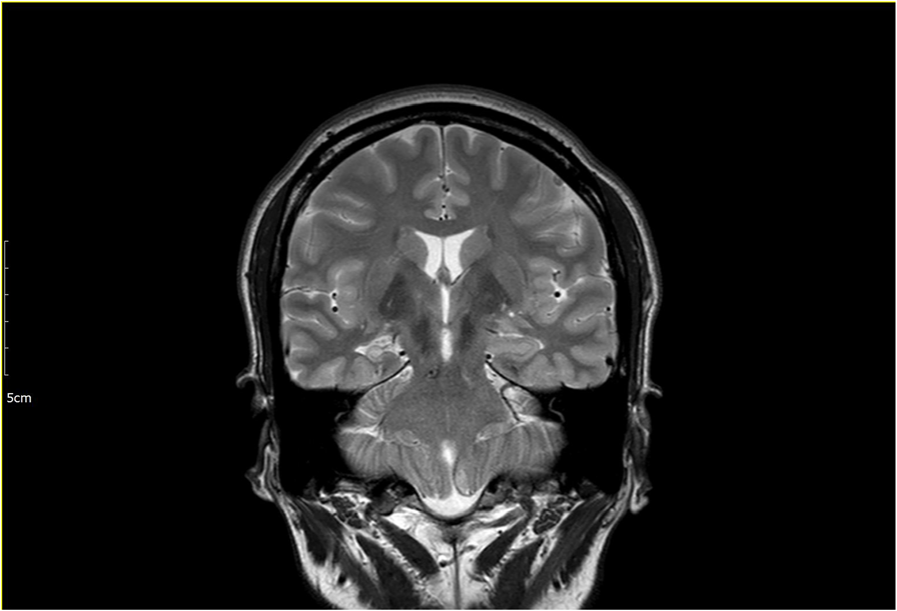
Magnetic Resonance Imaging. T2-weighted oblique coronal magnetic resonance imaging revealed right hippocampal atrophy
